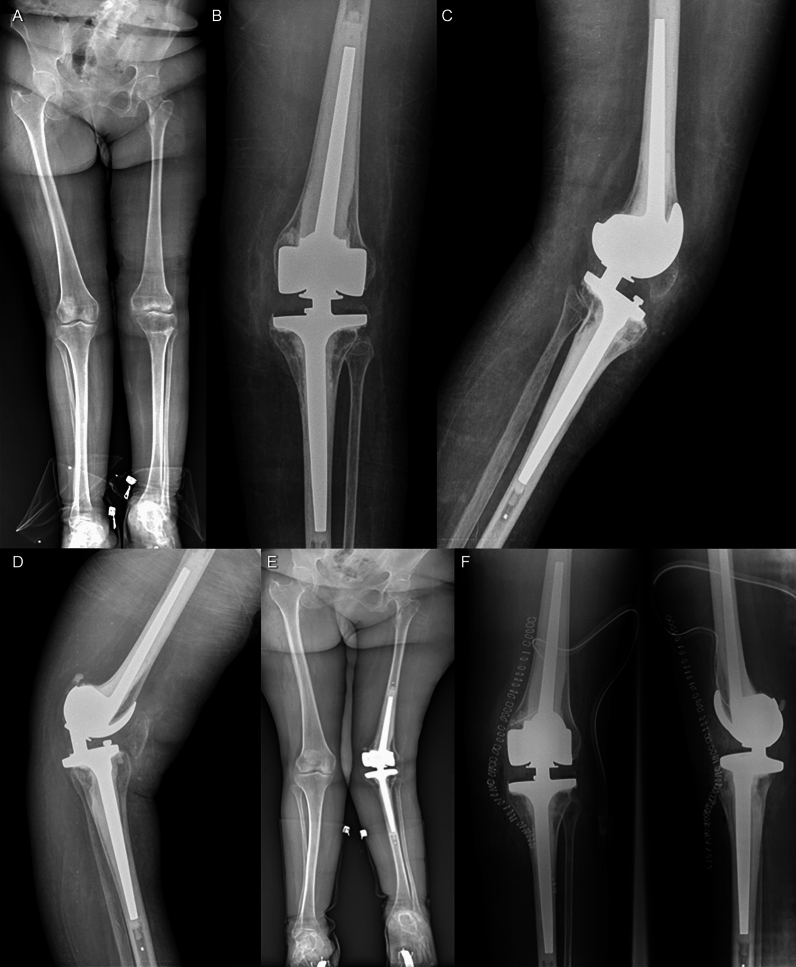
Case N.G.E. 66-year-old female; A The preoperative full weight-bearing X-ray shows a radiographic aspect of left knee osteoarthritis marked on the medial side. B–C 1-month postoperative left knee anteroposterior and latero-lateral X-ray showing the good positioning of the implant. D 1-year postoperative left knee anteroposterior X-ray showing the recurrence of hyperextension. E 1-year postoperative full weight-bearing X-ray shows the postoperative left knee valgus deformity. F Postoperative anteroposterior and latero-lateral left knee X-ray shows the final result after the substitution of the bush and the polyethylene insert
